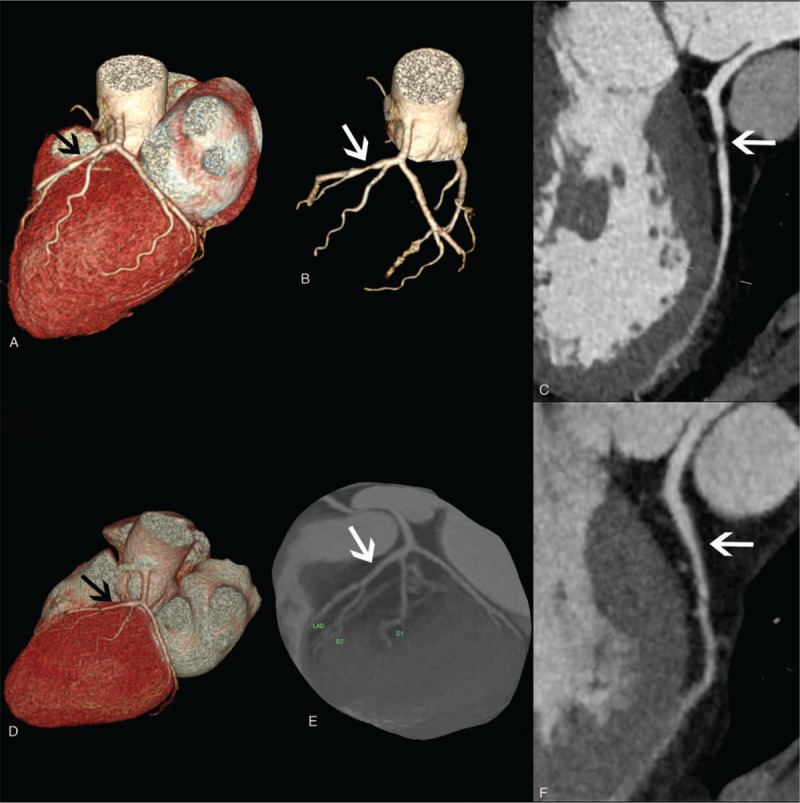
(A, B, C) Coronary angiography revealed 60% stenosis of the proximal left anterior descending coronary artery (arrows). (D, E, F) Coronary computed tomographic angiography revealed no stenosis in the proximal left anterior descending coronary artery (arrows).
